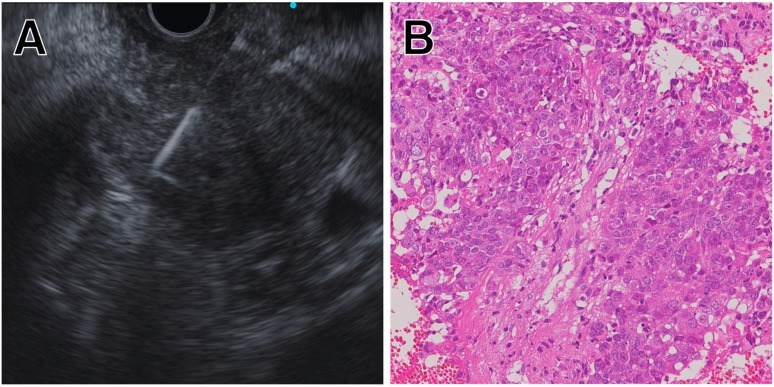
Representative case of Endoscopic Ultrasound-Guided Tissue Acquisition (EUS-TA) for pancreatic cancer (PC). (A) Endoscopic ultrasound-guided fine needle biopsy (EUS-FNB) of PC located in the body of the pancreas. (B) Tissue sample obtained by EUS-FNB showing large tissue fragments of poorly differentiated adenocarcinoma.
